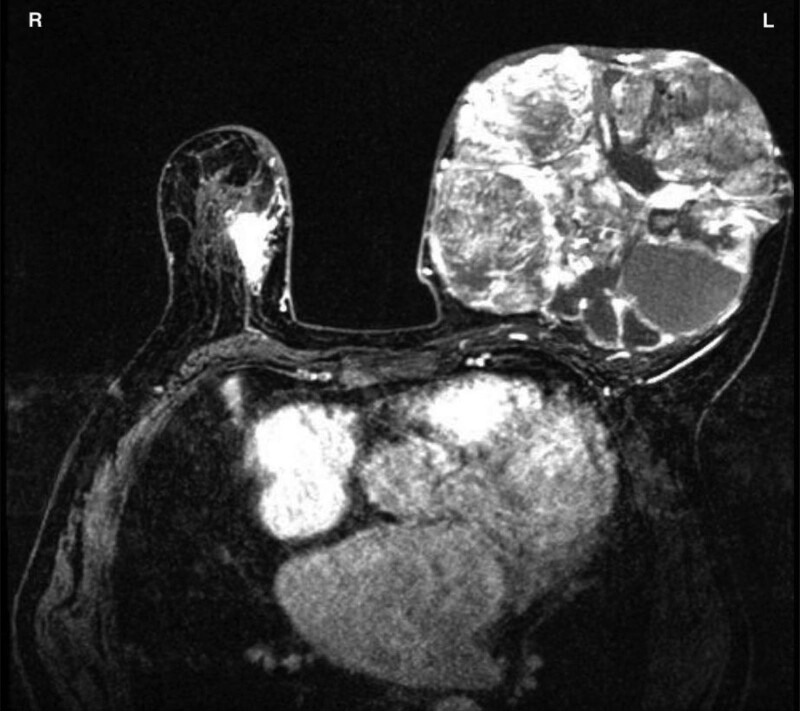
MRI of the breasts revealed a big mass in the left breast and a smaller lump in the right.
